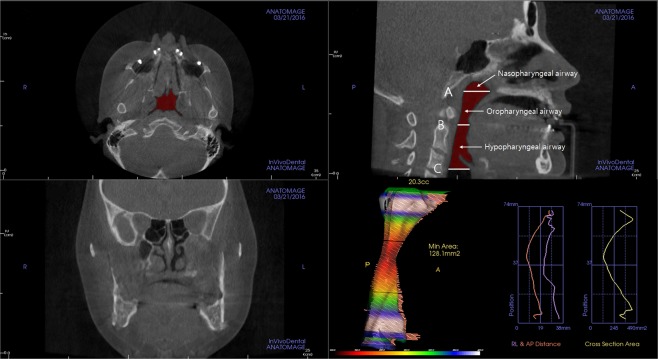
Three-dimensional measurement of the volume of PAS and reference planes and the division of pharyngeal airway space for volumetric measurement. Cone beam computed tomography data were imported to the program for visualizing pharyngeal airway space (PAS) and measuring the volume of PAS. Reference planes were set as follow: (A), posterior nasal spine (PNS) plane (axial plane passing through the PNS); (B), uvula plane (axial plane passing through the tip of the uvula); (C), epiglottis plane (axial plane passing through the base of the epiglottis). The pharyngeal airway was divided into three spaces based on the reference planes: the nasopharyngeal airway (between the highest point of the upper airway and the PNS plane), the oropharyngeal airway (between the PNS plane and the uvula plane), and the hypopharyngeal airway (between the uvula plane and the epiglottis plane).
